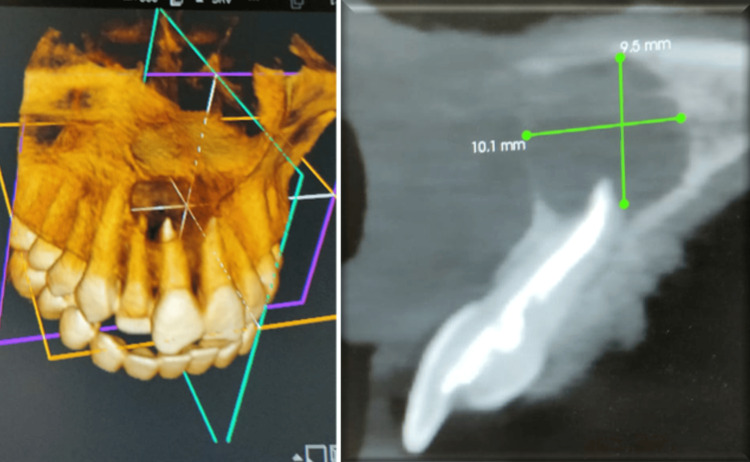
CBCT examination CBCT: cone-beam computed tomography.
